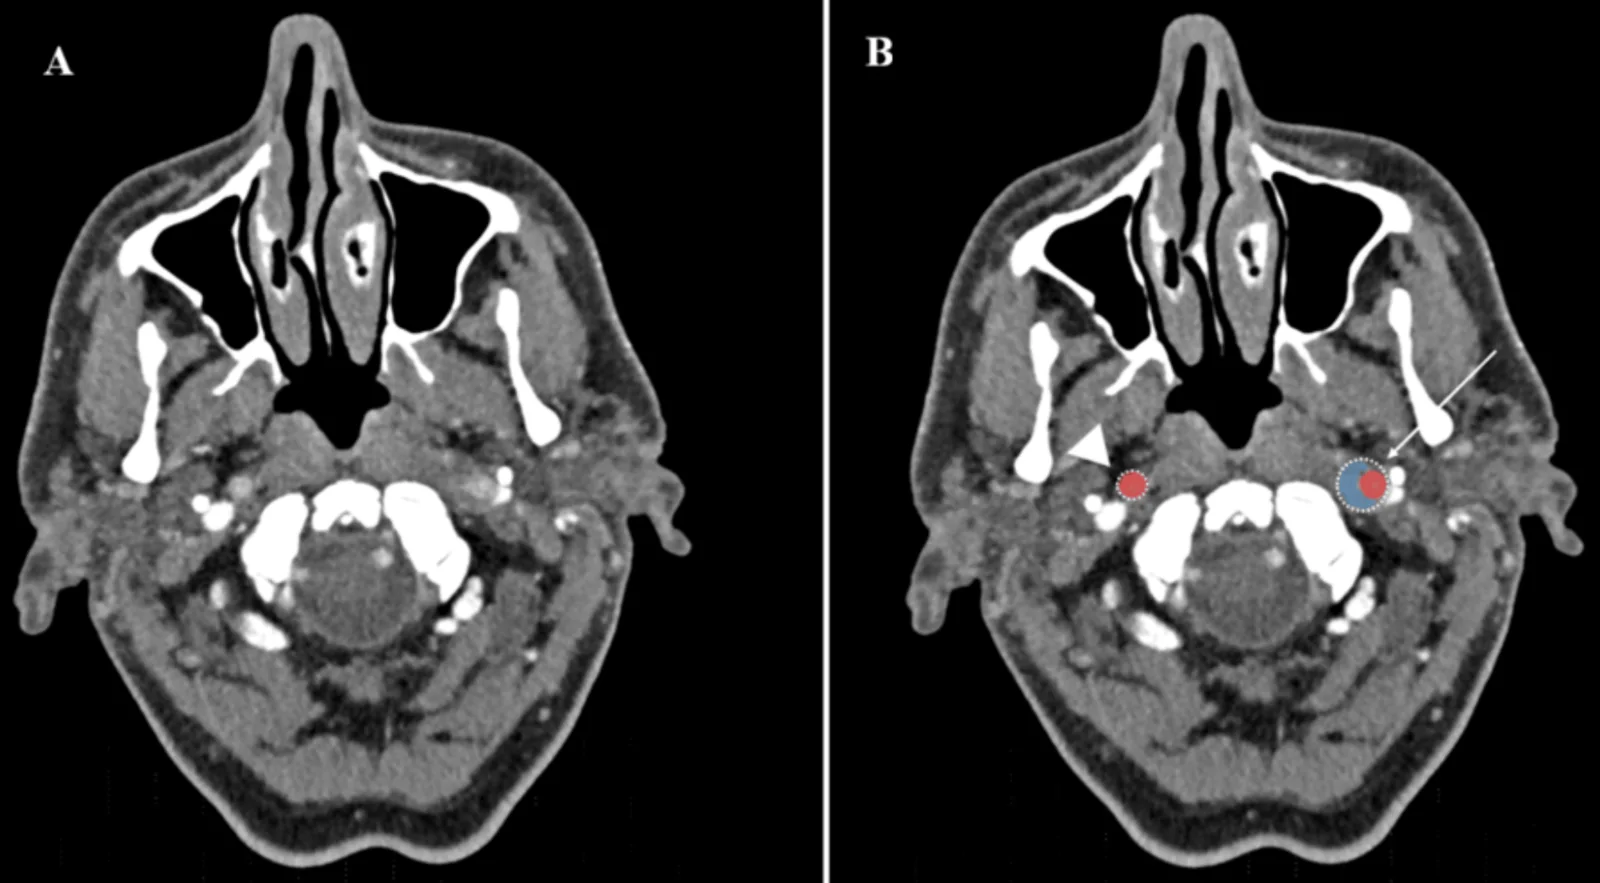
Axial CTA (A) and annotated image (B) at a level below the skull base showing the distal right (arrowhead) and left (arrow) internal carotid arteries. The left ICA (dotted line) is expanded due to the presence of an intramural hematoma (blue area); however, only minimal luminal stenosis is observed (red circle) CTA: computed tomography angiography; ICA: internal carotid artery
